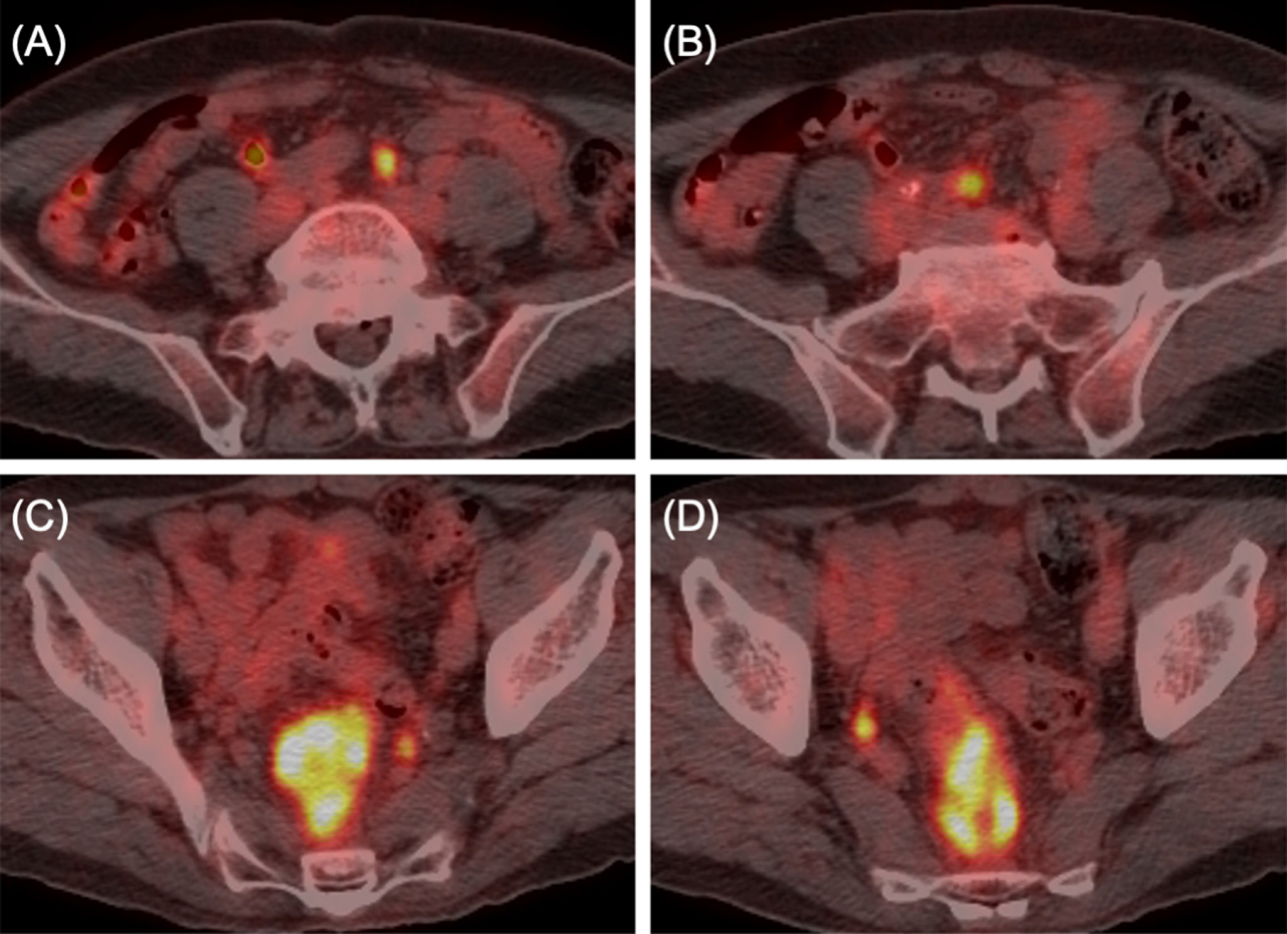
Positron emission tomography‐computed tomography images. Uptake of ^18^F‐fluorodeoxyglucose in multiple lateral lymph nodes identified in the bilateral common iliac regions (A, B) and bilateral internal iliac regions with accumulation in the tumor (C, D) before chemotherapy.
